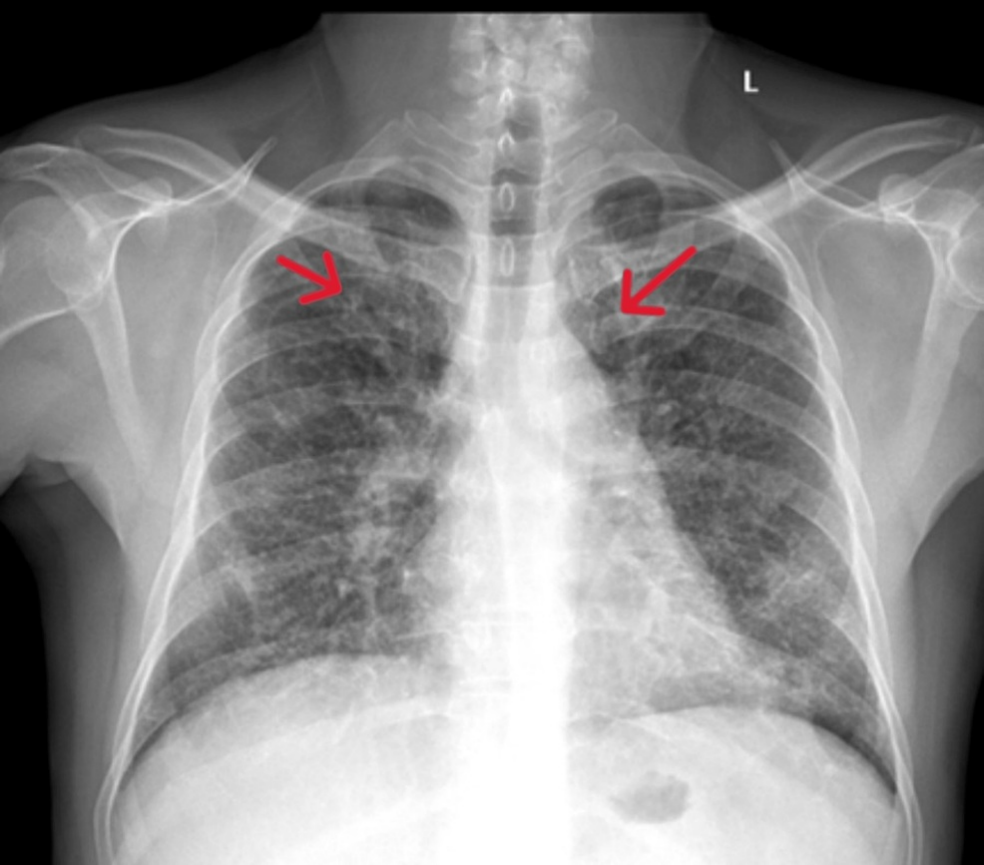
Chest X-ray showing bilateral pulmonary opacities on both upper lobes.
